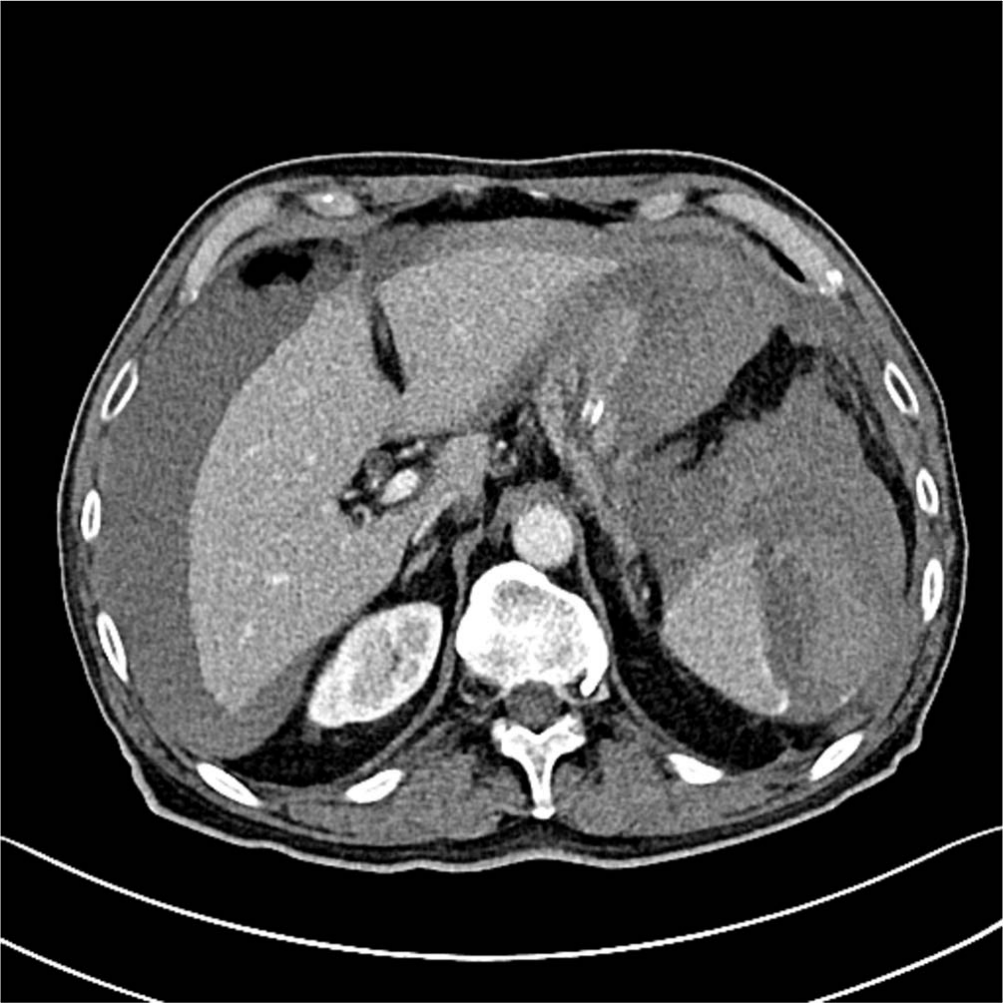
The CT scan reveals splenic hematoma with a significant blood halo surrounding the liver.
